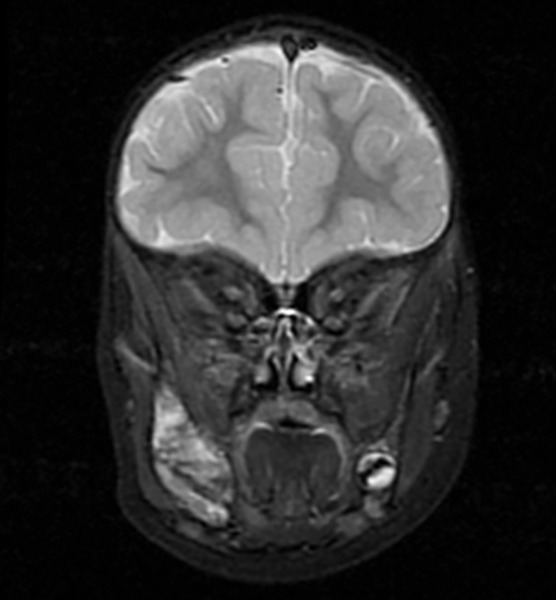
Magnetic resonance imaging scan showing soft tissue involvement
